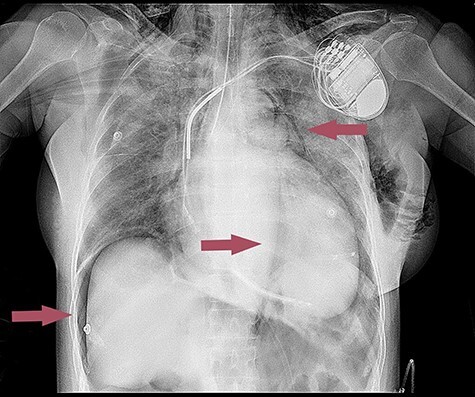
Chest X-ray after tracheal rupture.
